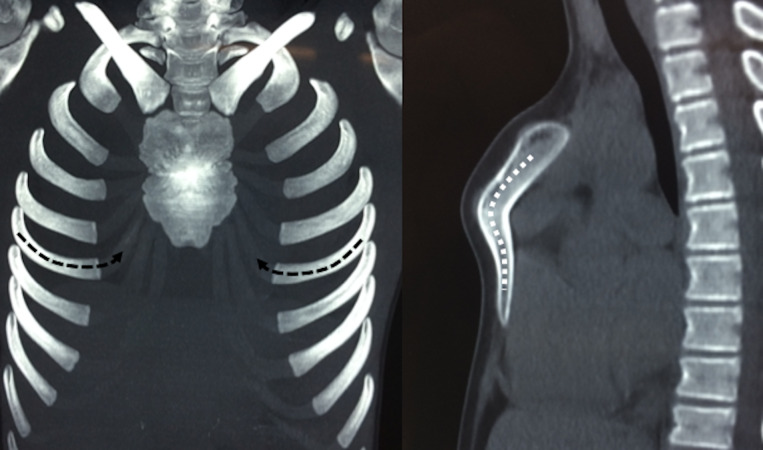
3D chest wall computed tomography scan of a patient with pectus arcuatum. This
